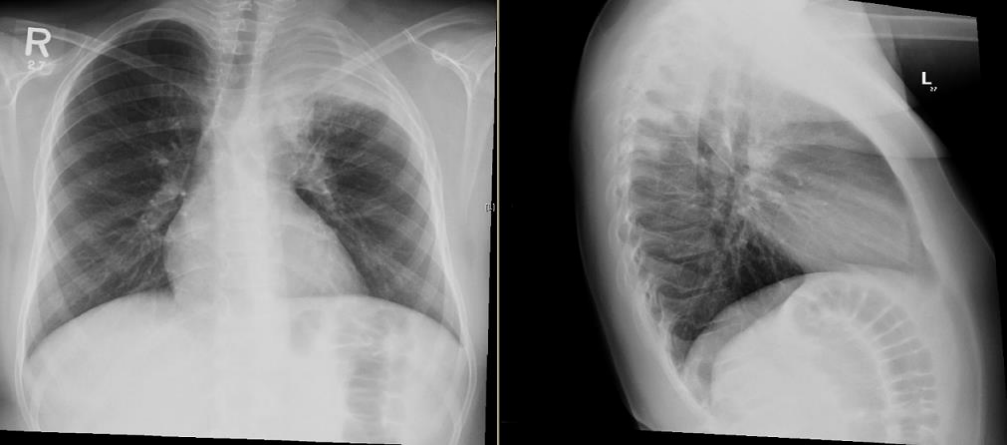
chest X-ray showed left perihilar and upper lobe opacity
